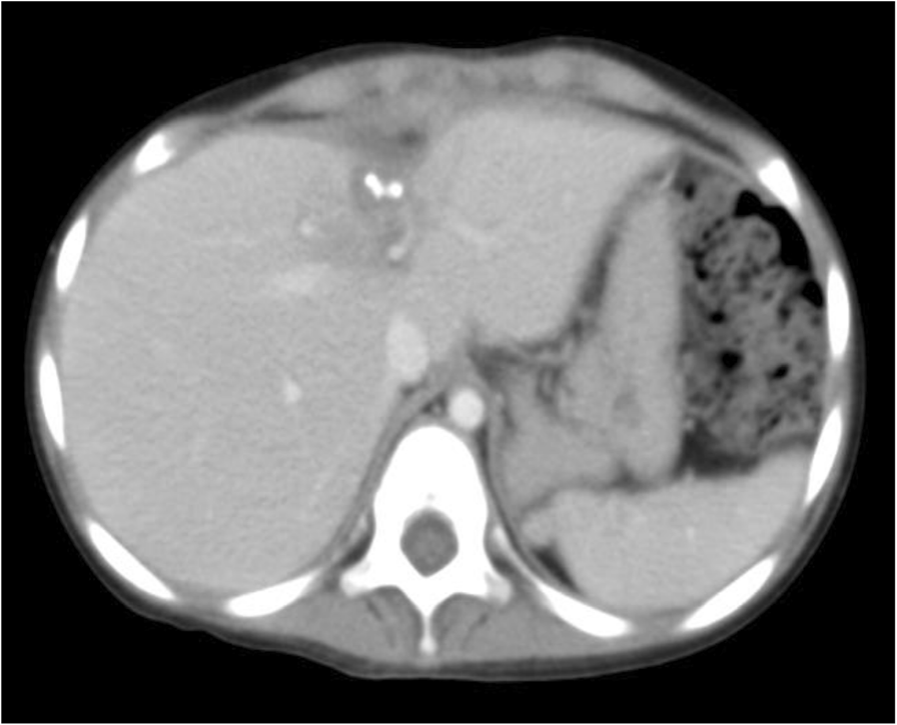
The tumor was well controlled on CT images at 2 months after IORT
